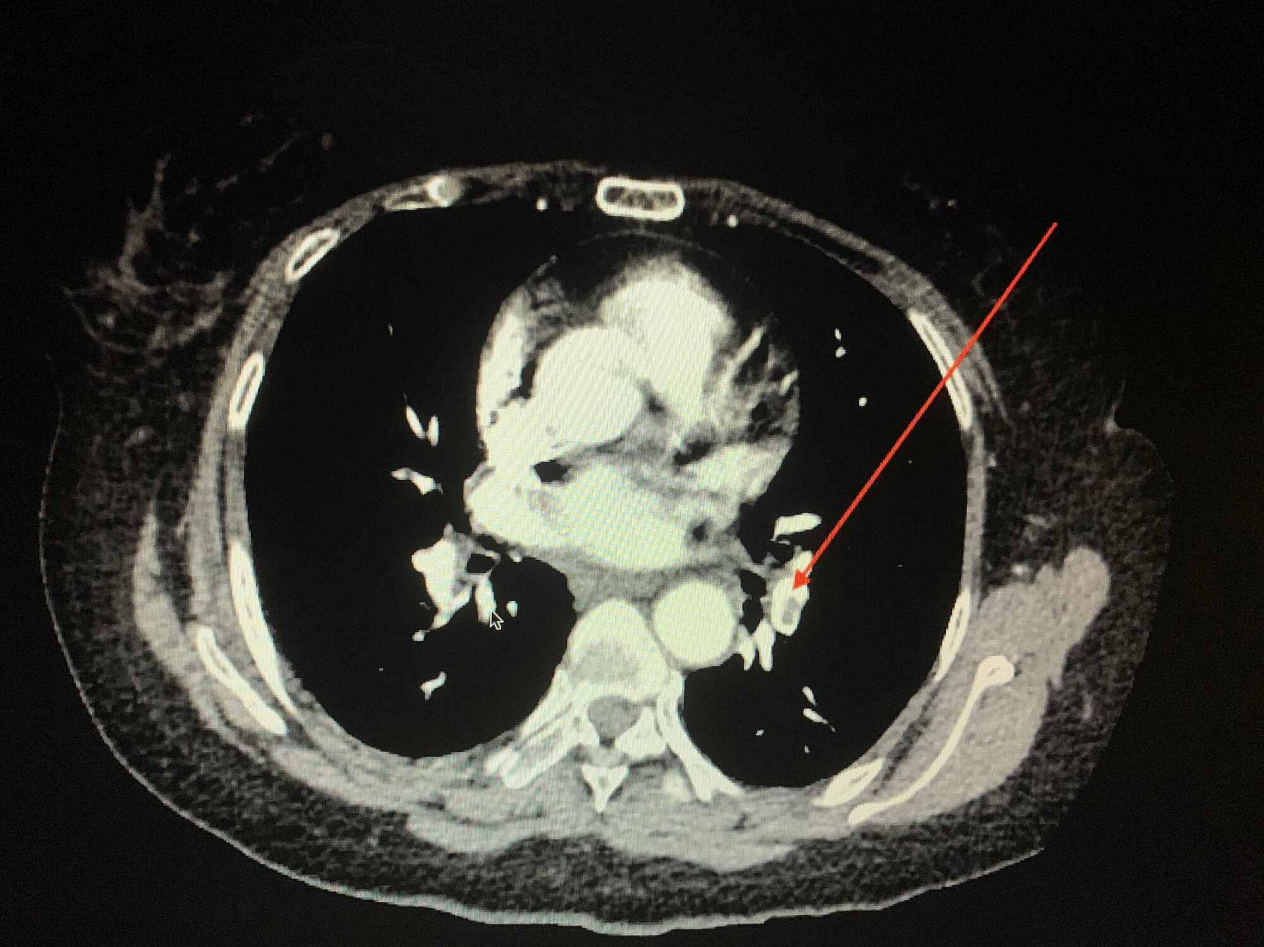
CT angiogram chest pulmonary embolism (PE) study demonstrating a small linear filling defect
